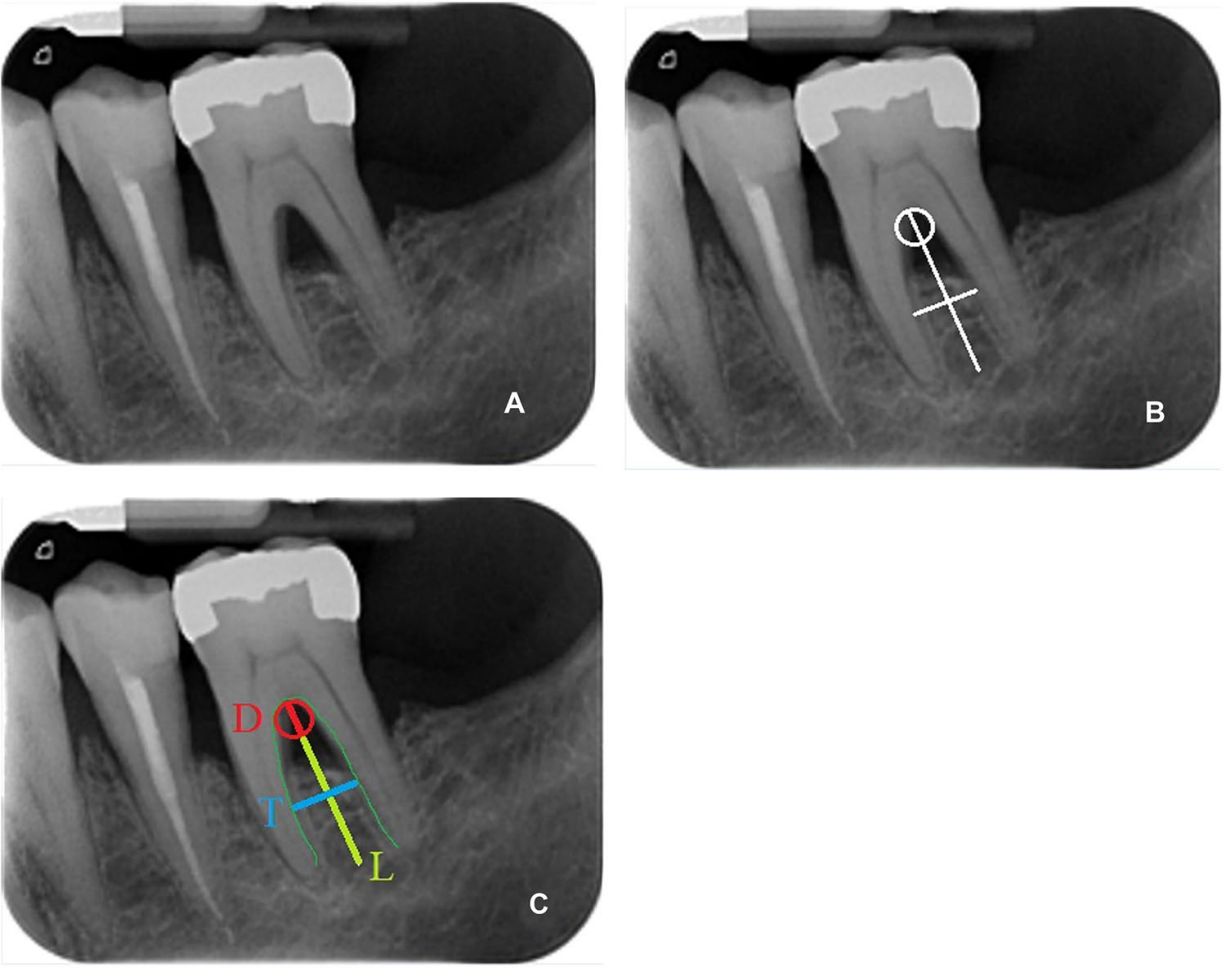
Example of root divergence assessment. A Peri-apical X-ray. B Application of the “visual” evaluation method for assessing root divergence. C) The osculating circle (D), the transversal width (T), and the longitudinal length (L) are depicted in the X-ray. RFW is obtained from the ratio of D and L, while IFW is obtained from the ratio of T and L. Picture from Limiroli et al. [24]
